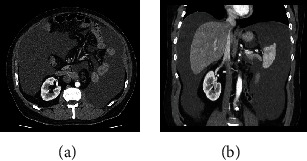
Computed tomography scan. Massive ascites can be seen in the (a) axial and (b) coronal sections.
